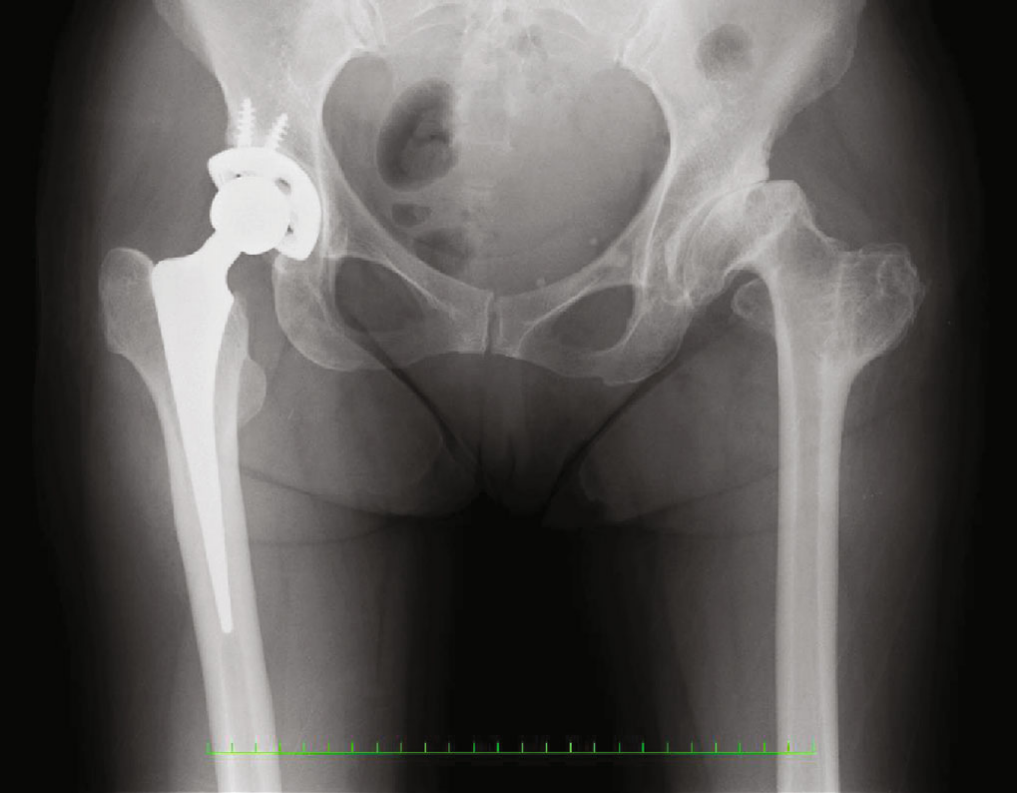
Radiographs of Case 2 before left total hip arthroplasty.
